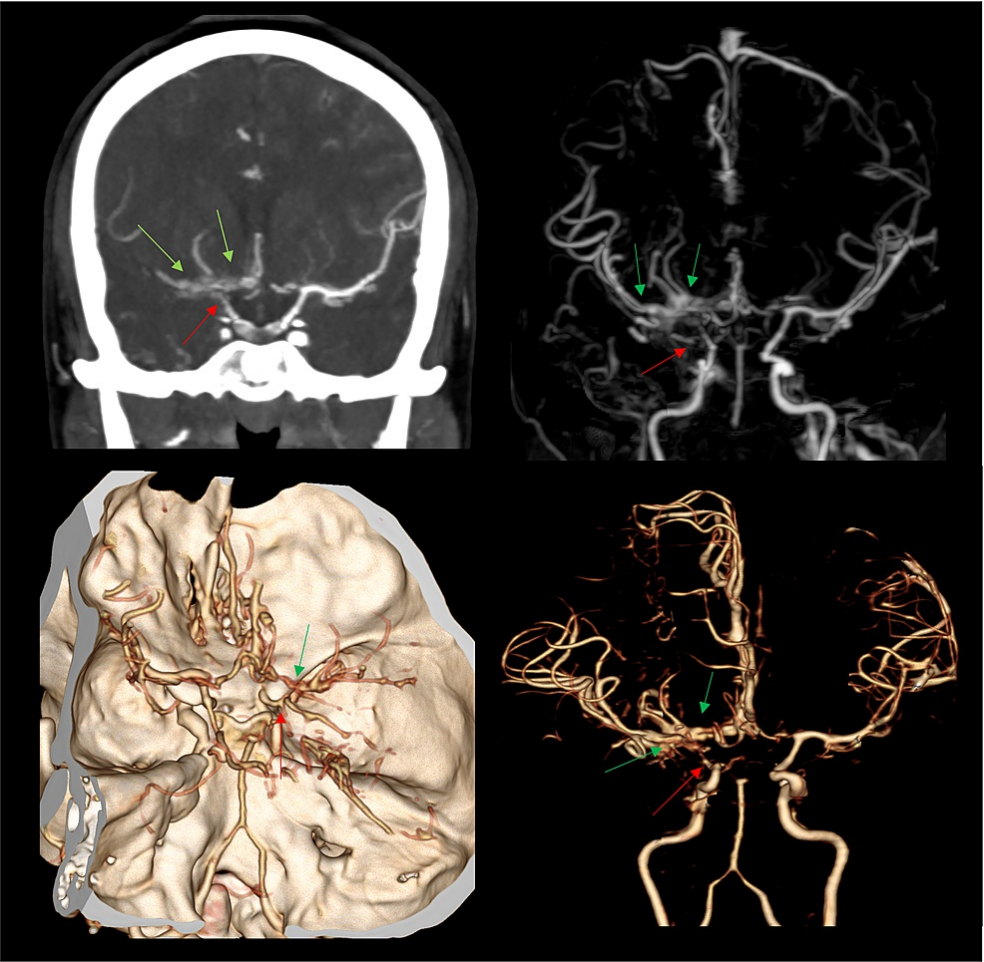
Classic complex collateralization on CTA images CTA demonstrating tapering and complete occlusion of right carotid terminus (red arrow) with multiple collateral arteries along the right carotid terminus, A1 and M1 segments (green arrows) compatible with MMD. CTA: computed tomography angiography; MMD: moyamoya disease
